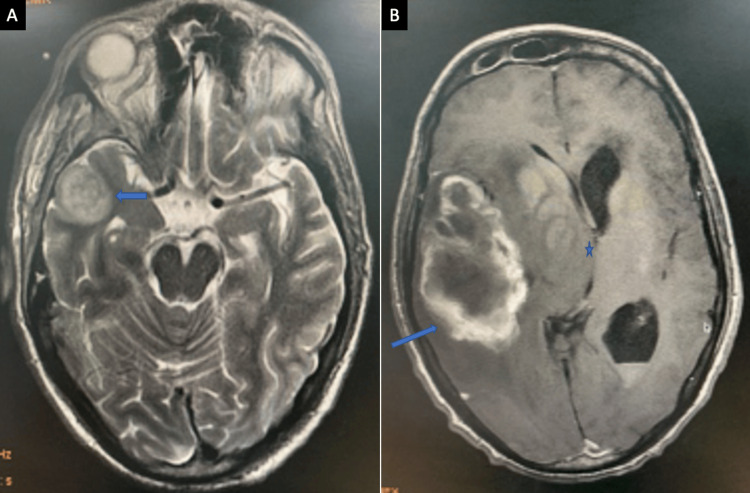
A case of acute invasive fungal infection with intracranial extension displaying a large intra-axial T2 heterogeneous signal intensity, lesion in the right temporal lobe (↗) (MRI, T2 axial). (B) Lesion is displaying irregular peripheral enhancement (↗) and significant mass effect in the form of effacement of adjacent sulci and right lateral ventricle along with midline shift toward the left side (*) (MRI, post-contrast T1 axial). MRI: magnetic resonance imaging
